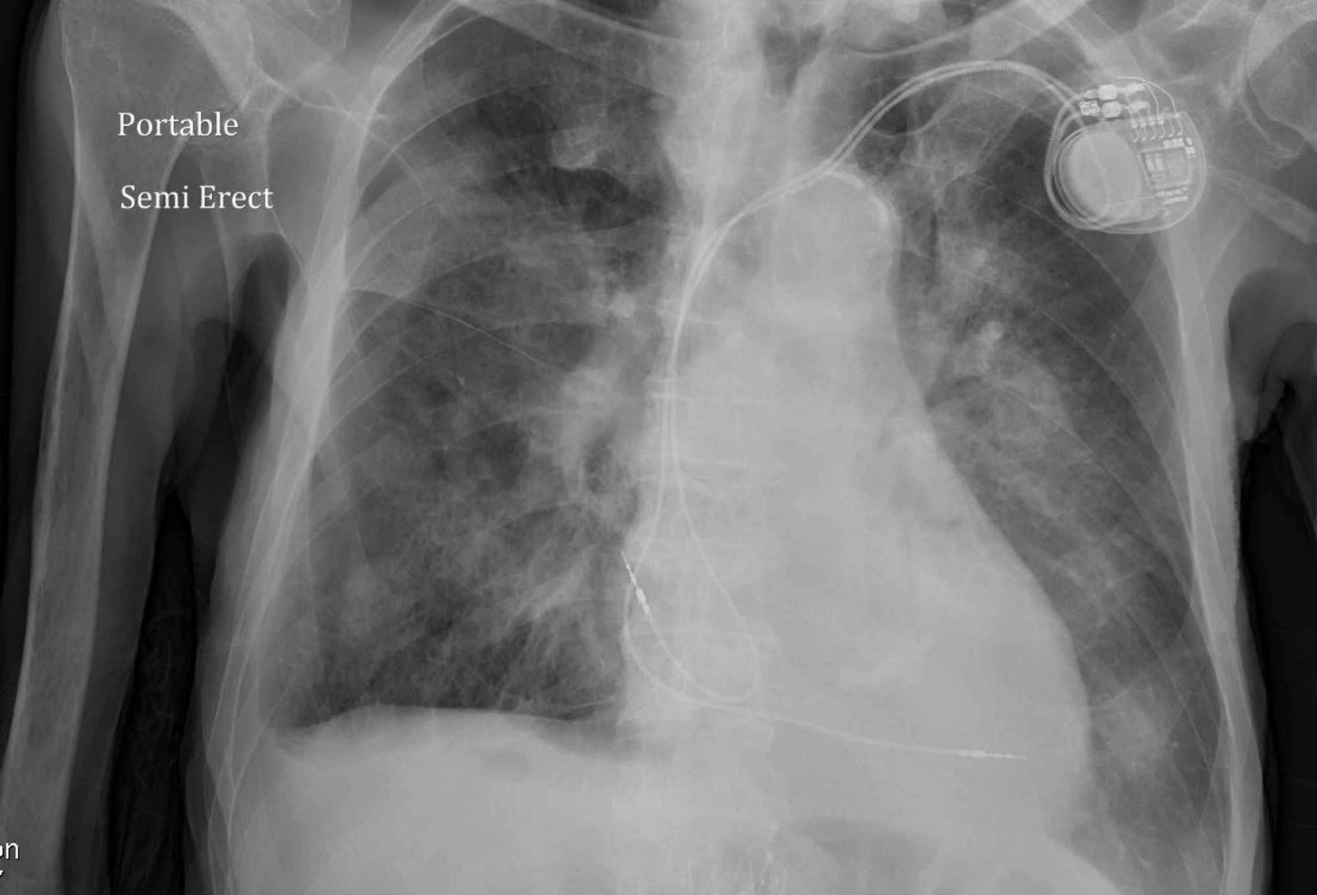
Chest X-ray showing bilateral lower lobe infiltrates
